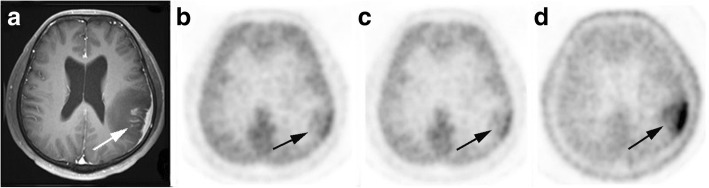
A patient aged 40–45 years with cerebral inflammatory lesion. Contrast enhanced MRI (a) shows the left frontal parietal lobe and adjacent meningeal lesion (arrow) was obvious heterogeneous enhanced. Significant higher uptake of ^11^C-MET (d) and mild increased uptake of ^18^F-FDG (b) and ^13^N-NH_3_ (c) were observed
